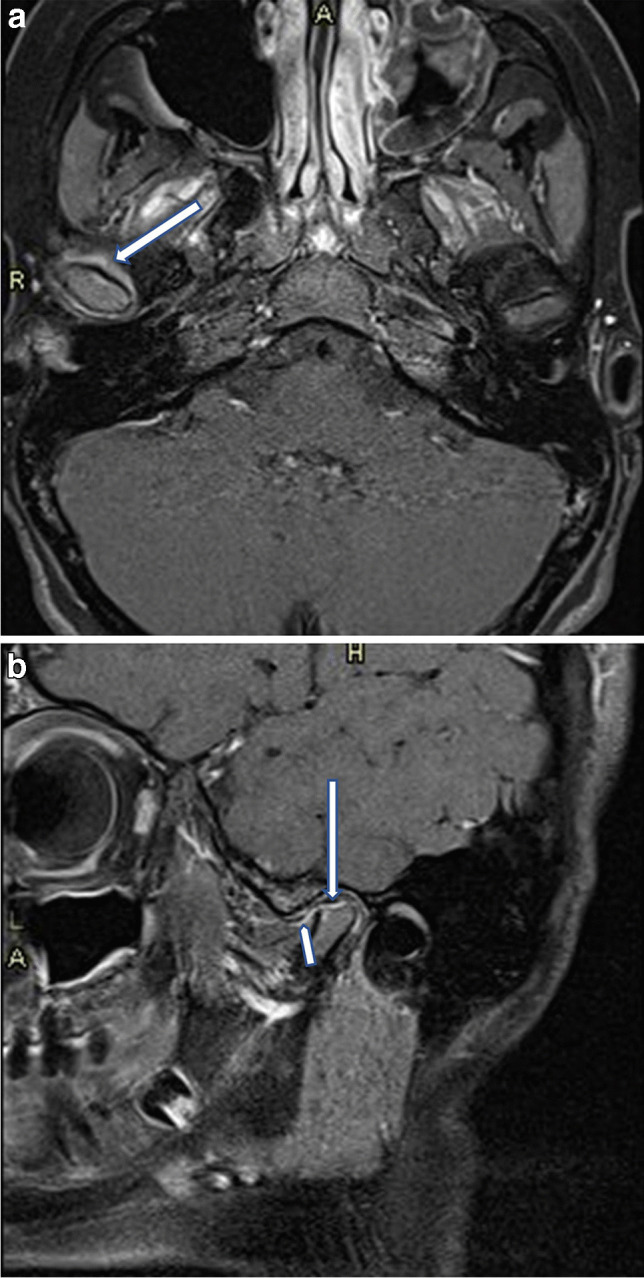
Postcontrast T1-weighted fat-suppressed magnetic resonance images of the temporomandibular joints in a 14-year-old girl with oligoarticular juvenile idiopathic arthritis (ANA positive). a Axial image shows synovial thickening and enhancement on the right (*arrow*)—a sign of active synovitis. b Sagittal image shows a flattened mandibular head (*long arrow*) and synovial thickening and enhancement on the right (*short arrow*)—signs of chronic bone changes and active synovitis
